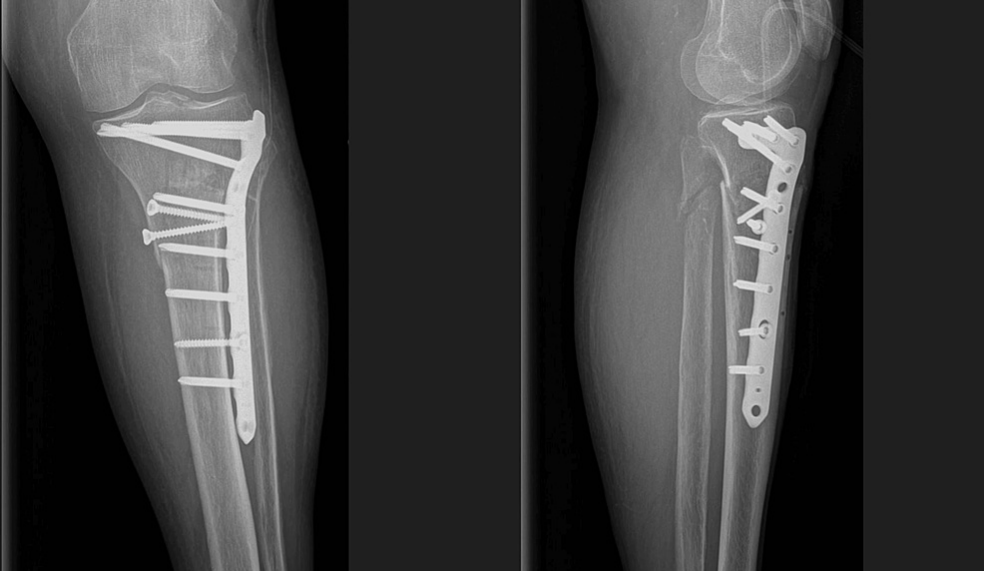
X-ray of the left proximal tibia showing anteroposterior and lateral views.
